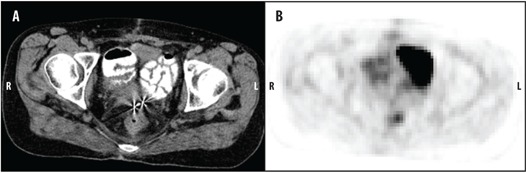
Postradiotherapy CT (a) and PET image (b) of the pelvis obtained four months following radiotherapy shows near complete resolution of tumor.
